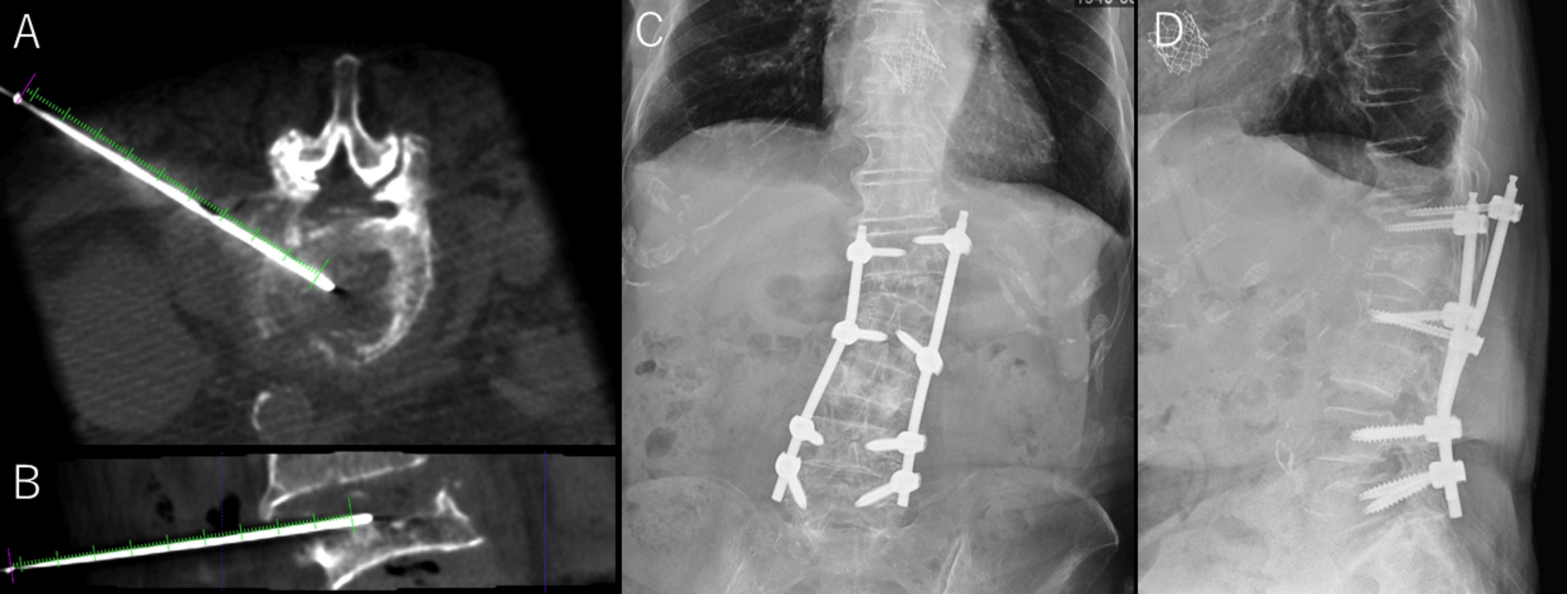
Surgical Intervention for Pyogenic Spondylitis CT-guided disc aspiration was performed at the L2/3 level. Axial (A) and sagittal (B) images confirm the needle reaching the intervertebral disc space. Postoperative radiographs demonstrate posterior fixation using percutaneous pedicle screws at the twelfth thoracic, second, fourth, and fifth lumbar vertebrae (C: anteroposterior view, D: lateral view).
